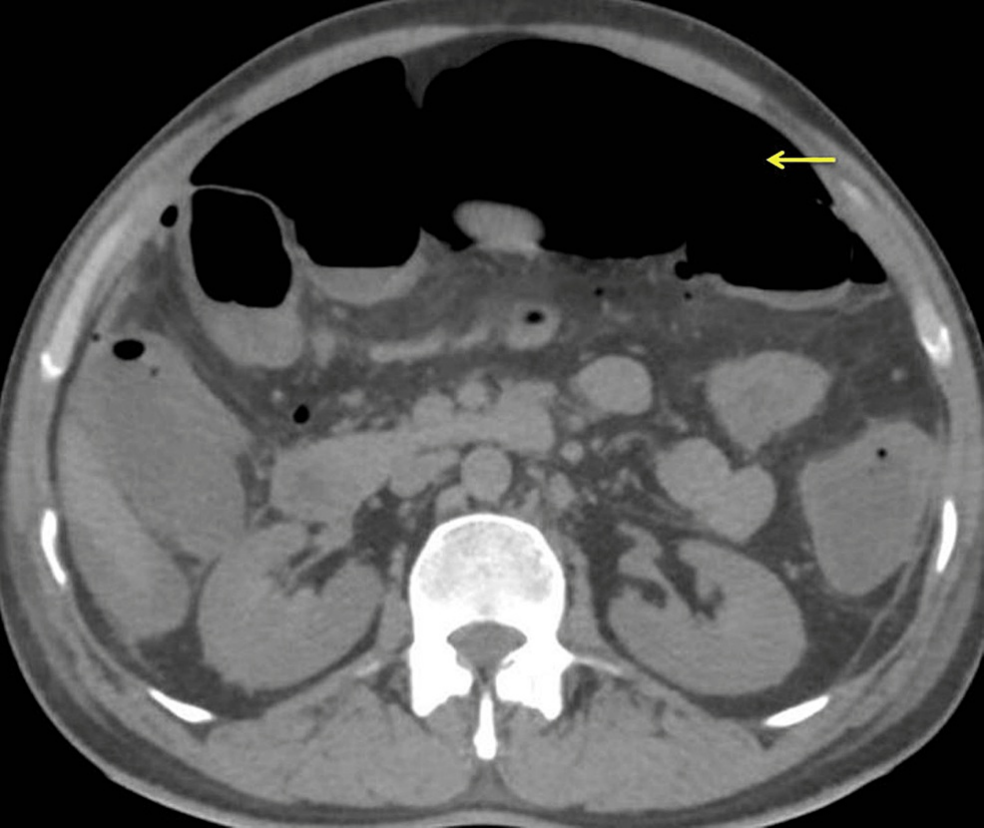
Computed tomography (axial view) showing massive pneumoperitoneum (yellow arrow).
